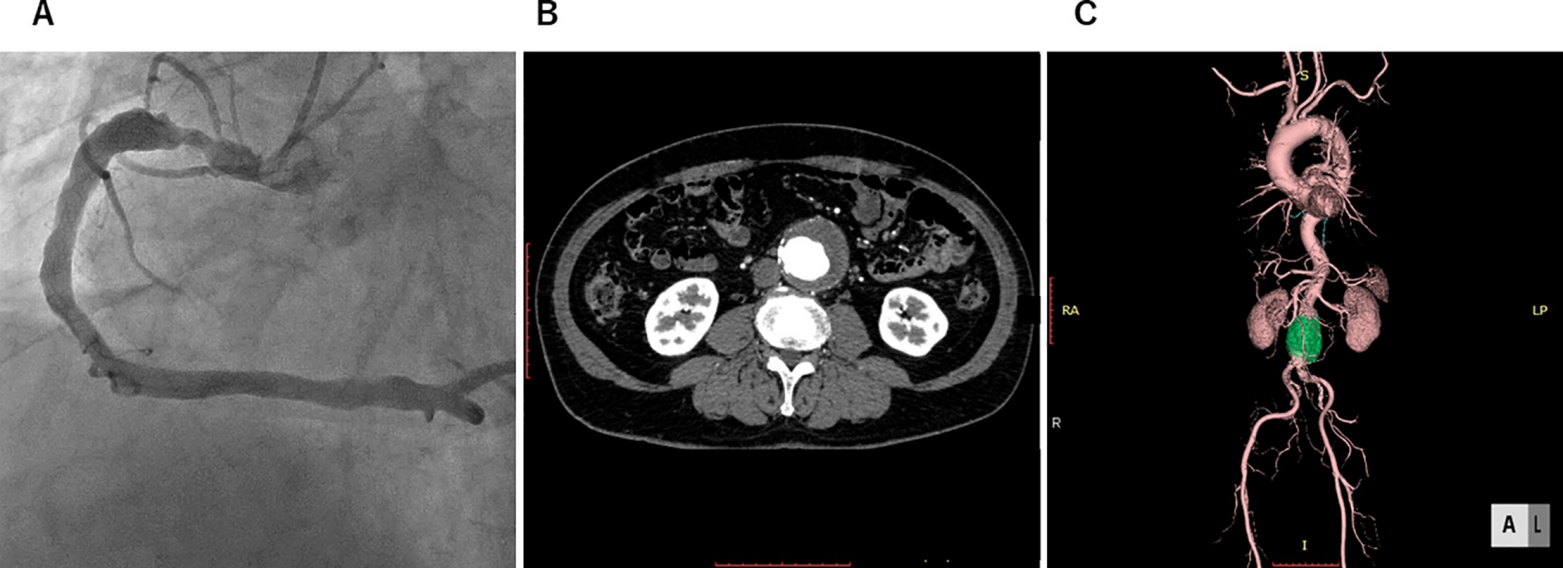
A representative case of coronary artery ectasia. Images of a 62-year-old man with an abdominal aortic aneurysm. Diffuse ectasia and focal stenosis in the right coronary artery are seen (A). The axial image (B) and 3D reconstruction image (C) of computed tomography show an infra-renal aortic aneurysm.
